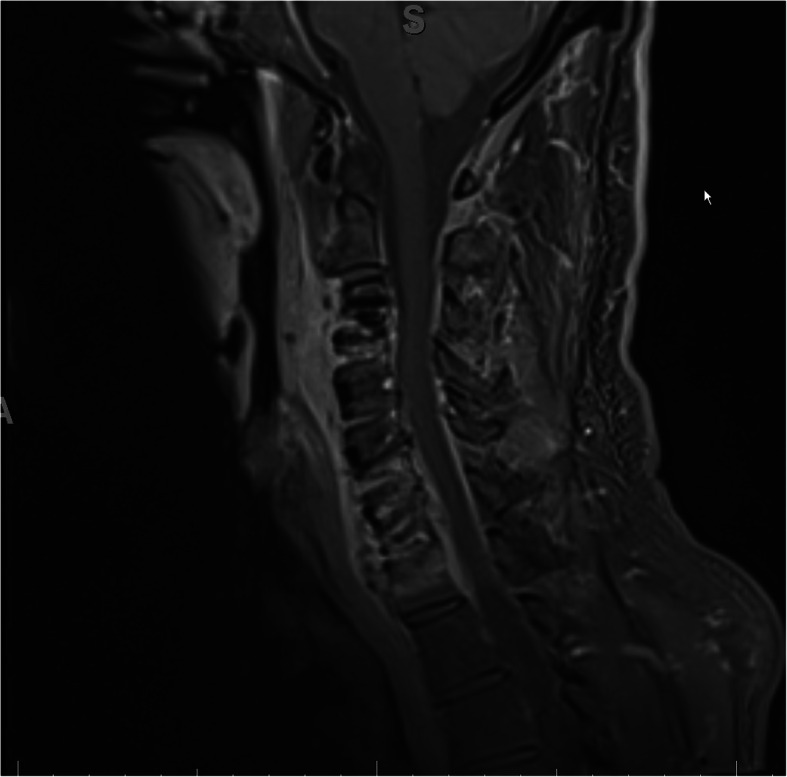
Sagittal MRI T1 fat suppression protocol with contrast performed 1 month after surgical drainage and antibiotic therapy
